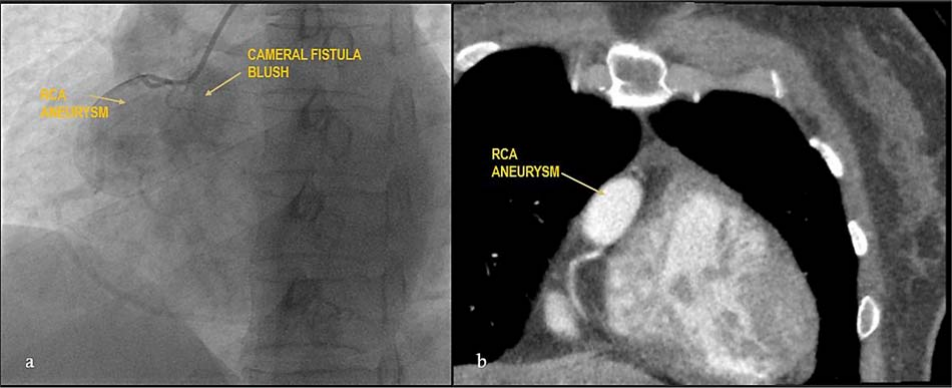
Giant RCA aneurysm with CCF. (a) CAG showing RCA aneurysm with RA blush; (b) RCA aneurysm in cardiac CT.
